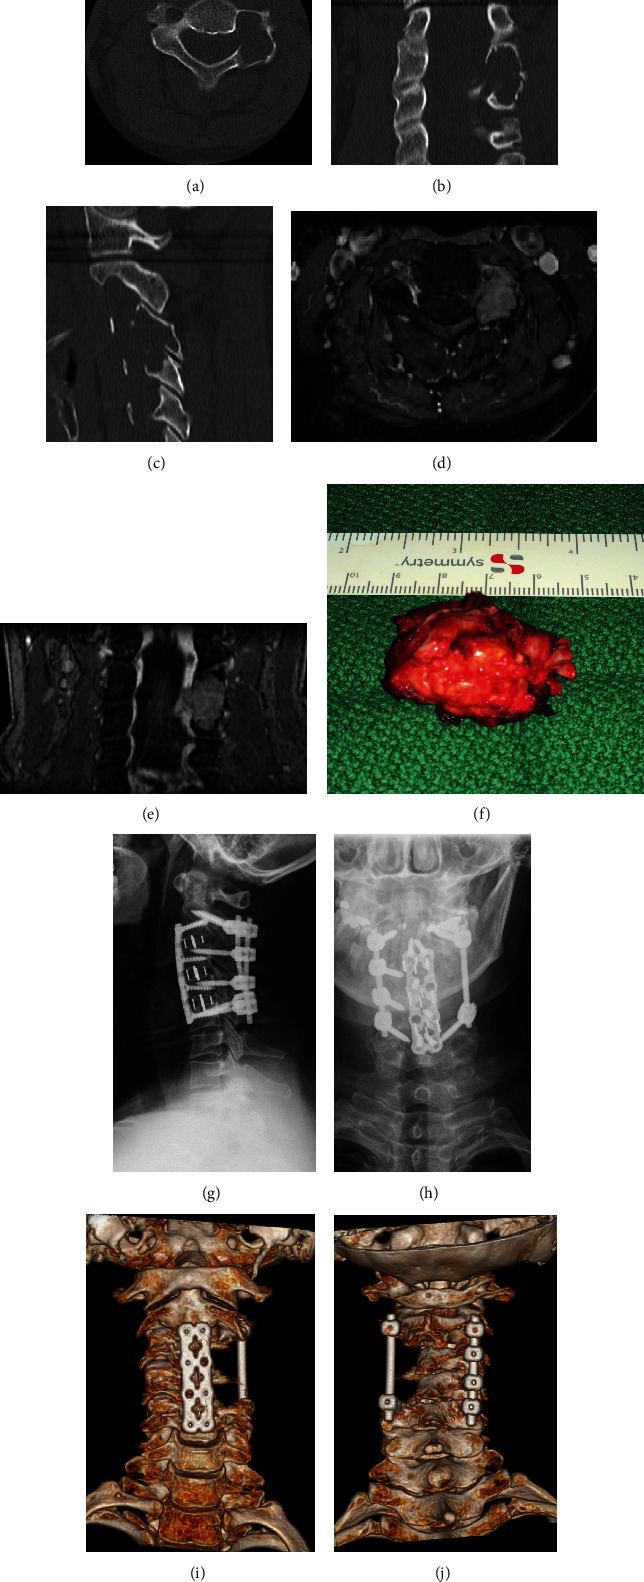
Case 1: aggressive osteoblastoma in C3/C4. (a–c) CT scan reconstructions of the cervical spine with a left-sided osteolysis in C3 and C4. (d, e) MRI shows the extent of the tumor and its close relation to the vertebral artery. (f) En bloc resected tumor. (g, h) Postoperative a.p. and lateral radiographs after resection of the tumor and combined posteroanterior stabilization. (i, j) CT scan 3-D reconstructions show the osseous defect after tumor resection.
